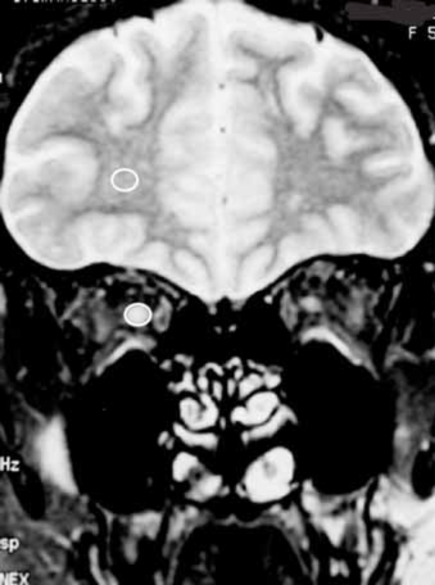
Signal intensity within white circle was measured in the one coronal MR image.
